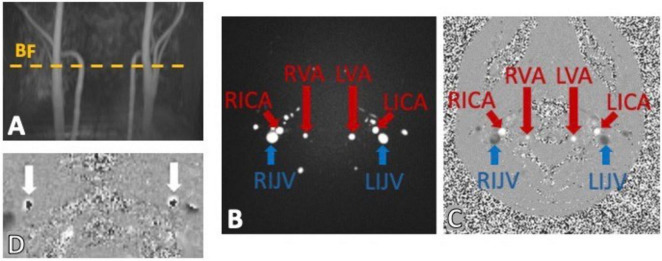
Example of phase contrast MRI (PC-MRI) sequence output using a time-of-flight (TOF) to place the imaging slice perpendicular to the main neck vessels (A). The output images have a magnitude (B) and phase component (C) encoding for the flows within the bilateral internal carotid arteries (RICA and LICA), vertebral arteries (RVA and LVA) and internal jugular veins (RIJV and LIJV). (D) Shows a classic example of velocity aliasing artifacts on a PC-MRI phase image. This commonly happens when a too low velocity encoding (VENC) is chosen and the fast spins appear to be flowing in the opposite direction (dark cores).
